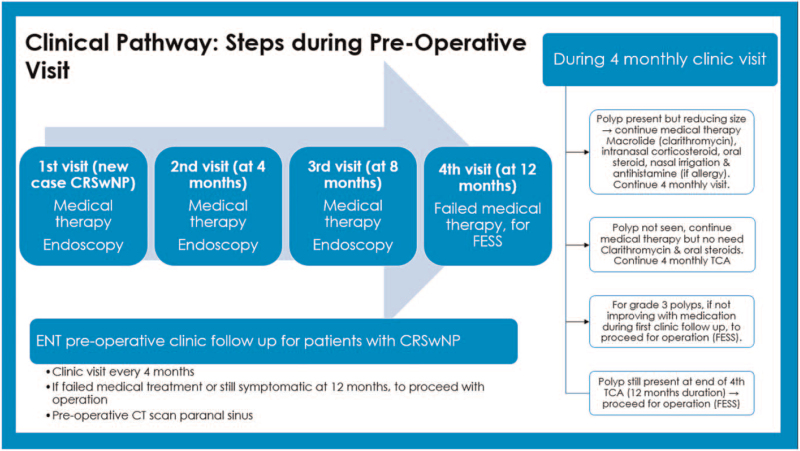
Clinical pathway: steps during preoperative visit. (FESS = functional endoscopic sinus surgery, CRSwNP = chronic rhinosinusitis with nasal polyposis, CT = computed tomography, TCA = to see again/ follow-up).
